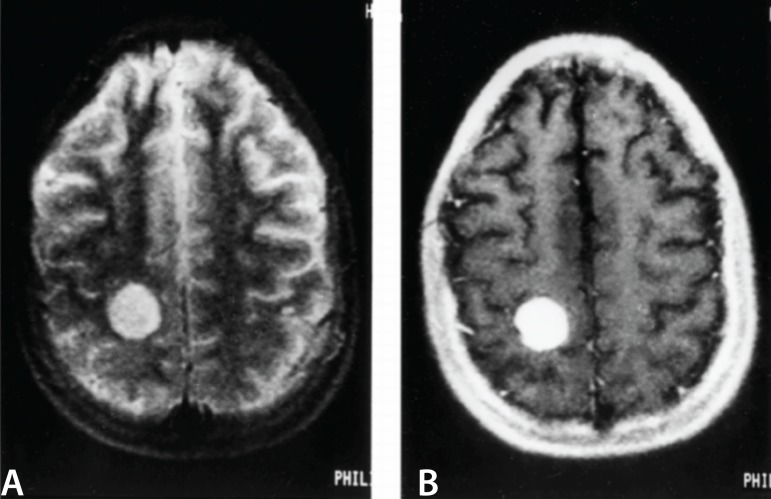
The lesion in the right parietal lobe hyperintense on the long MRI image
